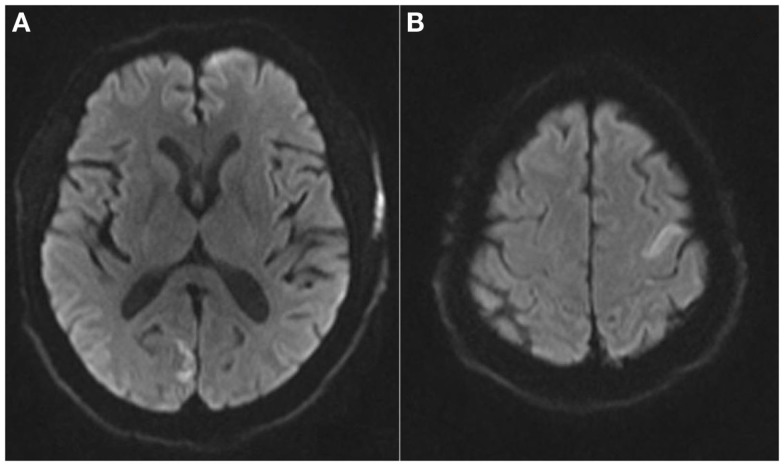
Magnetic resonance imaging brain axial diffusion weighted images demonstrating areas of restricted diffusion in the R occipital lobe (A) and left superior frontal lobe (B).
